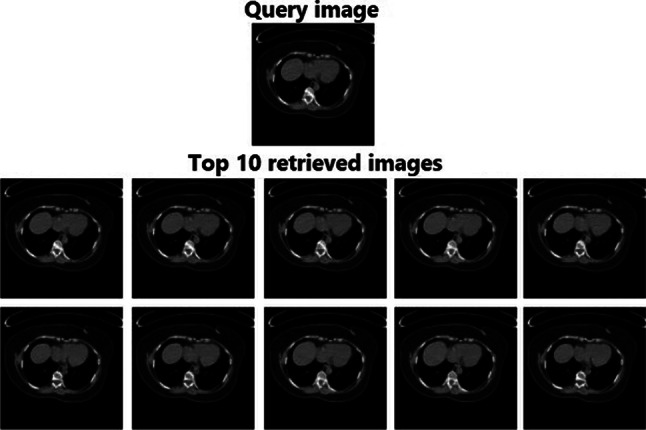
Retrieved images for a TCIA-CT database query using RbQE with VGG-19
